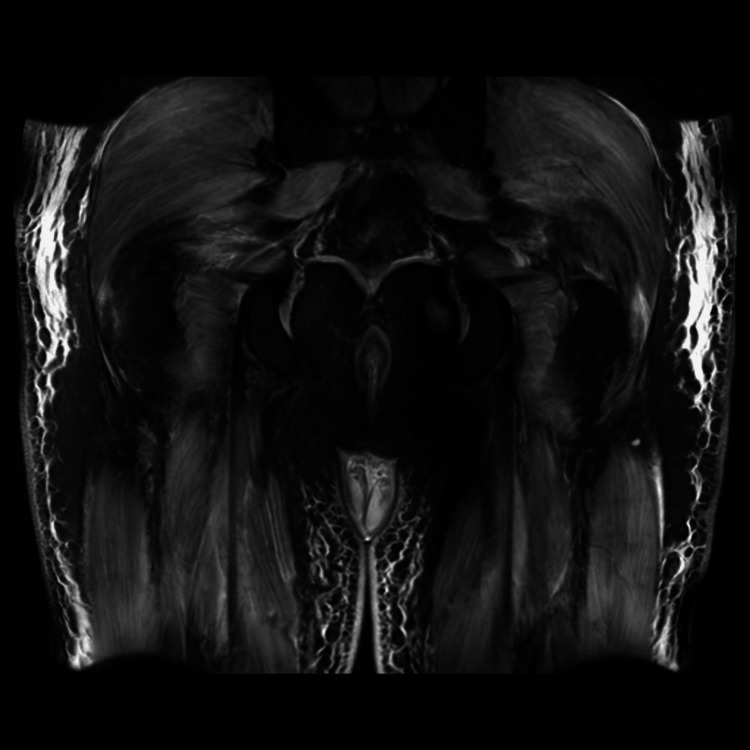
MRI pelvis showing extensive diffuse muscle oedema in both thighs and gluteal muscles.
